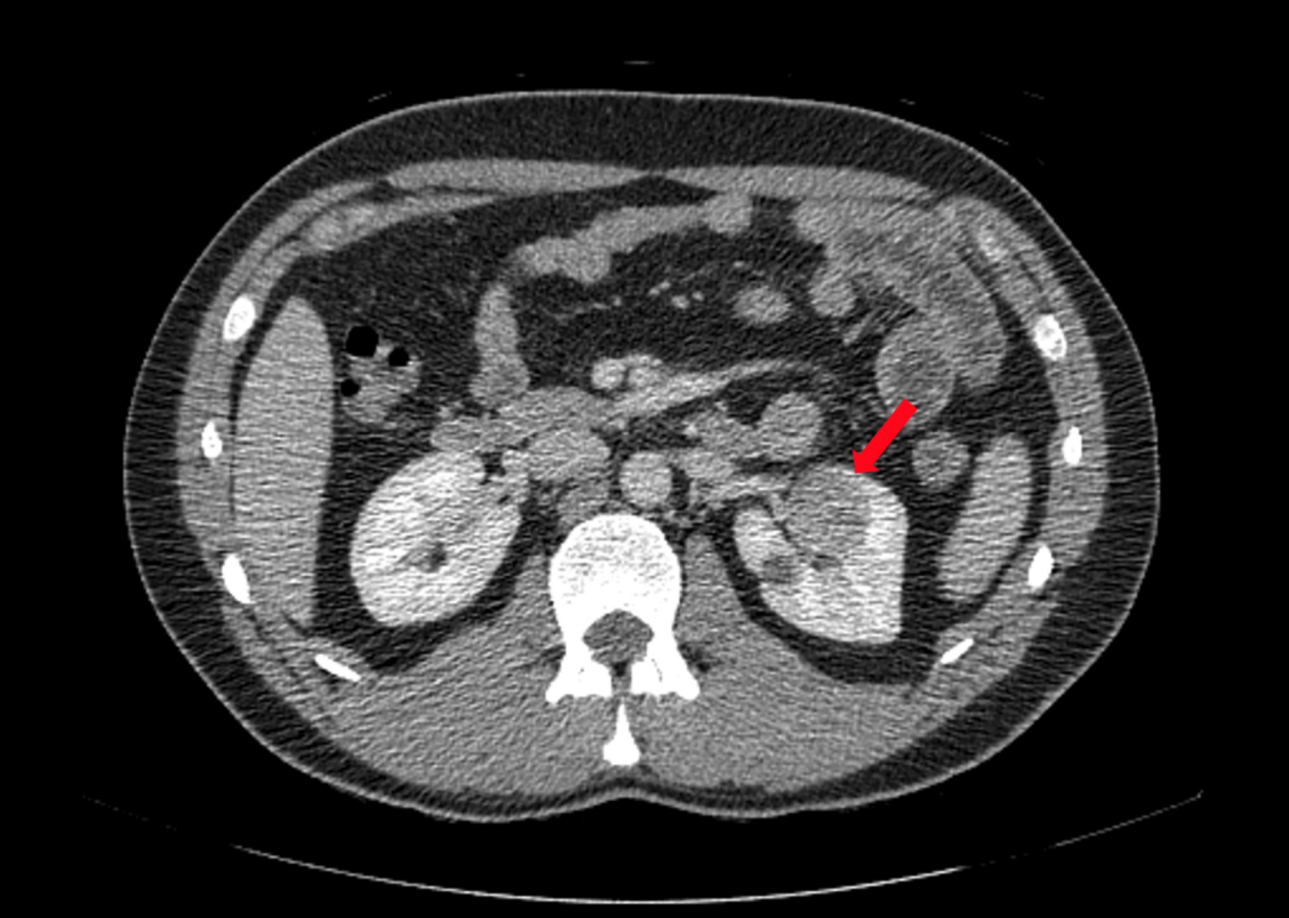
CT showing left renal mass (red arrow)
